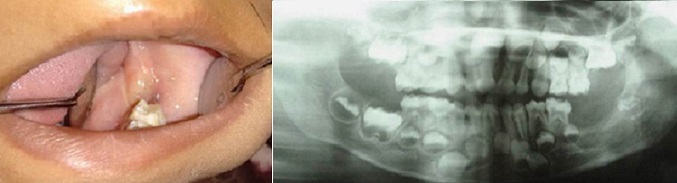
3 months post-operative clinical picture showing satisfactory healing & radiograph (OPG)-revealing partial filling of the surgical bone defect
